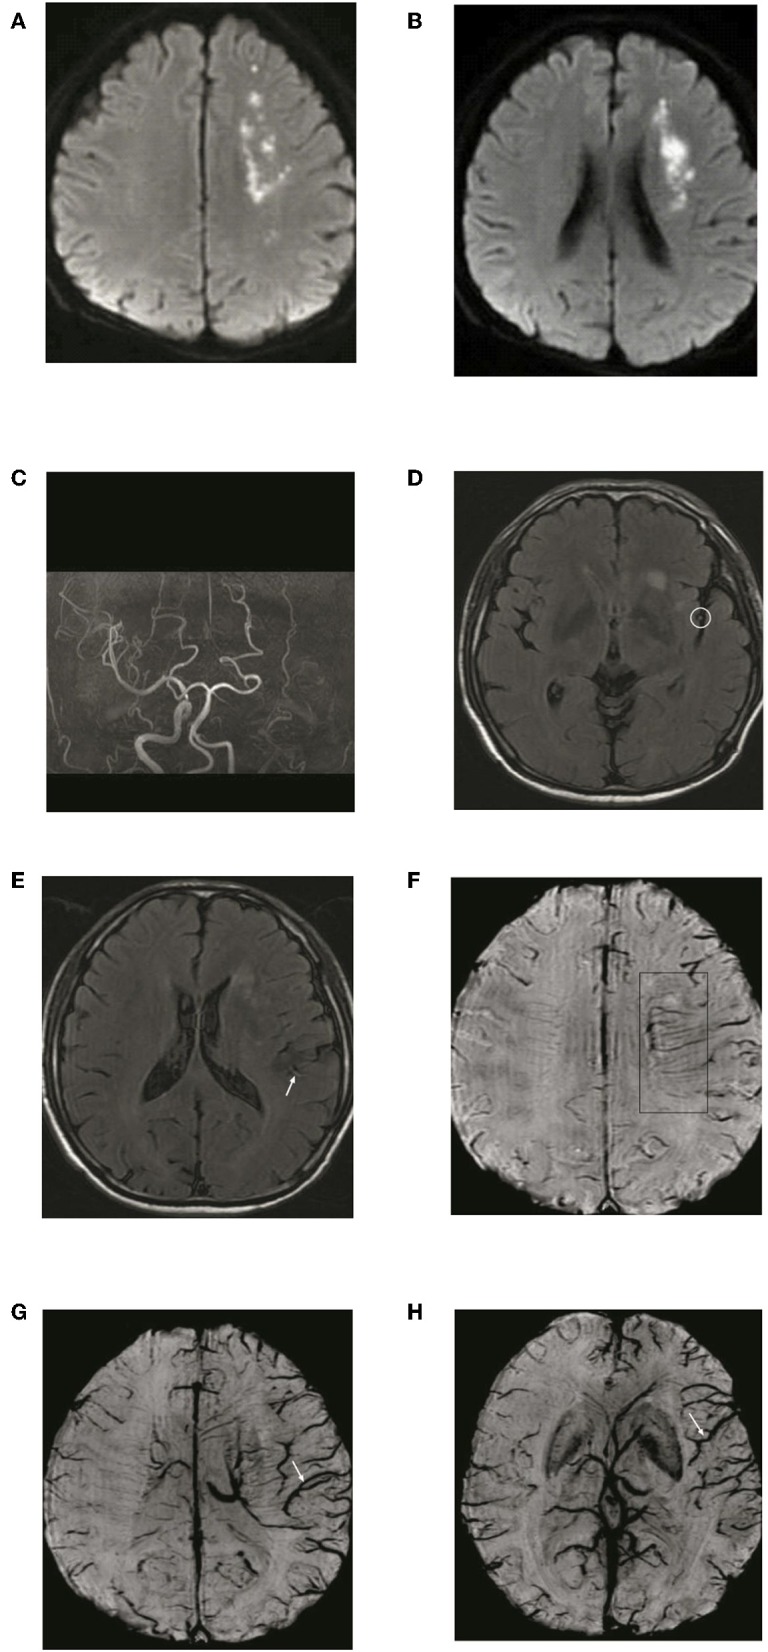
Typical patient with asymmetric deep medullary veins (ADMV). A 70-year-old male presented with right limb weakness for 6 h. (A,B) The left frontal lobe shows acute corona radiata cerebral infarction on DWI; (C) Occlusion of the left internal carotid artery on magnetic resonance angiography with maximal intensity projection; (D,E) HVS with a score of 2 on T2-FLAIR image: (F) Presence of ADMV; (G,H) ACV with a score of 6 on SWI.
